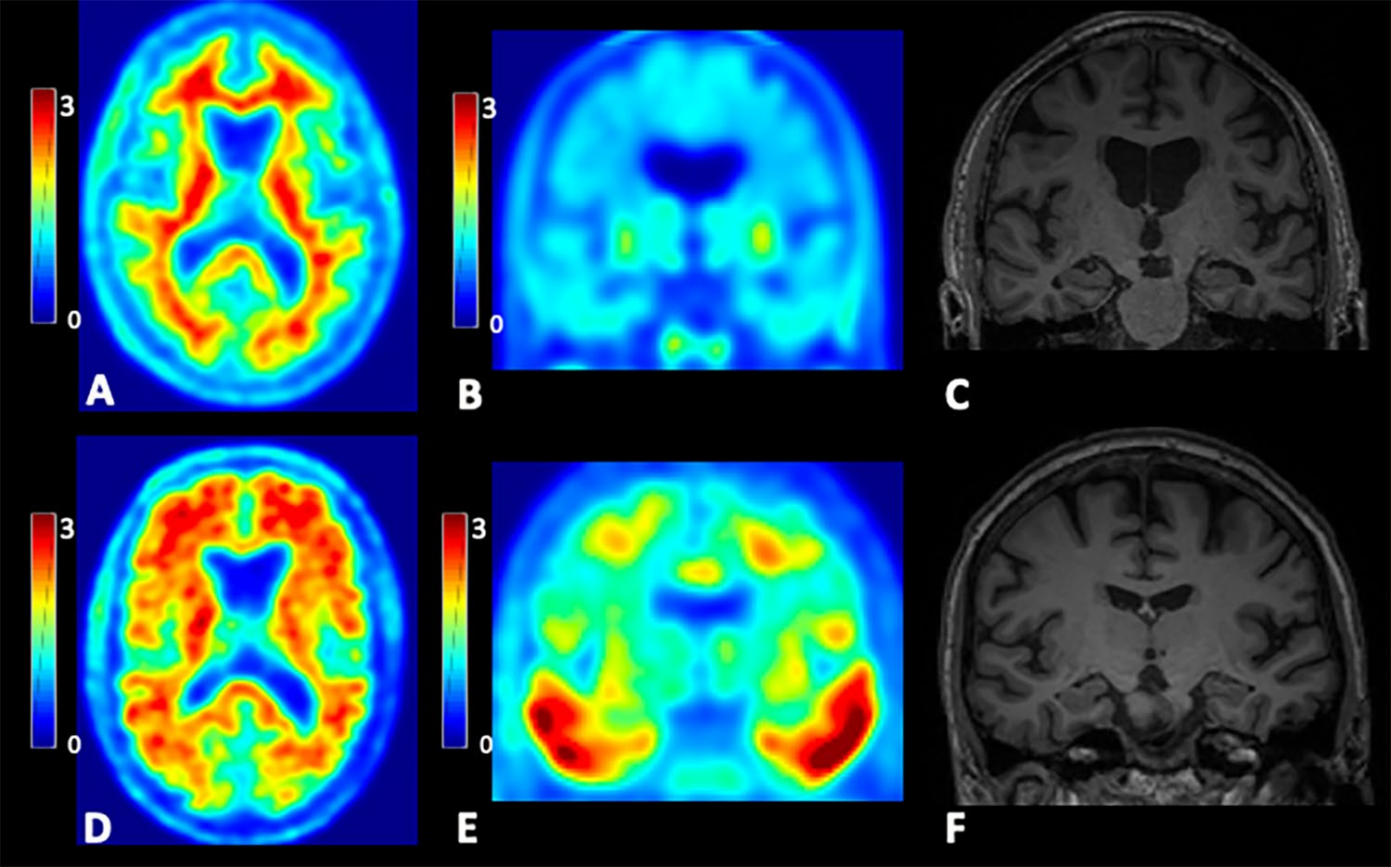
Axial ^18^F-florbetapir PET, coronal ^18^F-flortaucipir PET and coronal T1-weighted MR images from two subjects with mild cognitive impairment. SUVR images were intensity normalized by the cerebellar cortex. Subject 1 is a 75 year-old man with a global SUVR of 1.03 on ^18^F-florbetapir PET (A), consistent with a negative amyloid scan, a negative ^18^F-flortaucipir PET scan (B), and mild ventricular enlargement on MRI (C), suggestive of mild volume loss. His neutrophil-to-lymphocyte ratio (NLR) was 1.04, and his lymphocyte-to-monocyte ratio was 6.5. Subject 2 is a 74 year-old man with a global SUVR of 1.60 on ^18^F-florbetapir PET (D), consistent with a positive amyloid scan, a positive ^18^F-flortaucipir PET scan (E), and no significant volume loss on MRI (F). His NLR was 3.8, higher than Subject 1, and his LMR was 2.29, lower than Subject 1.
